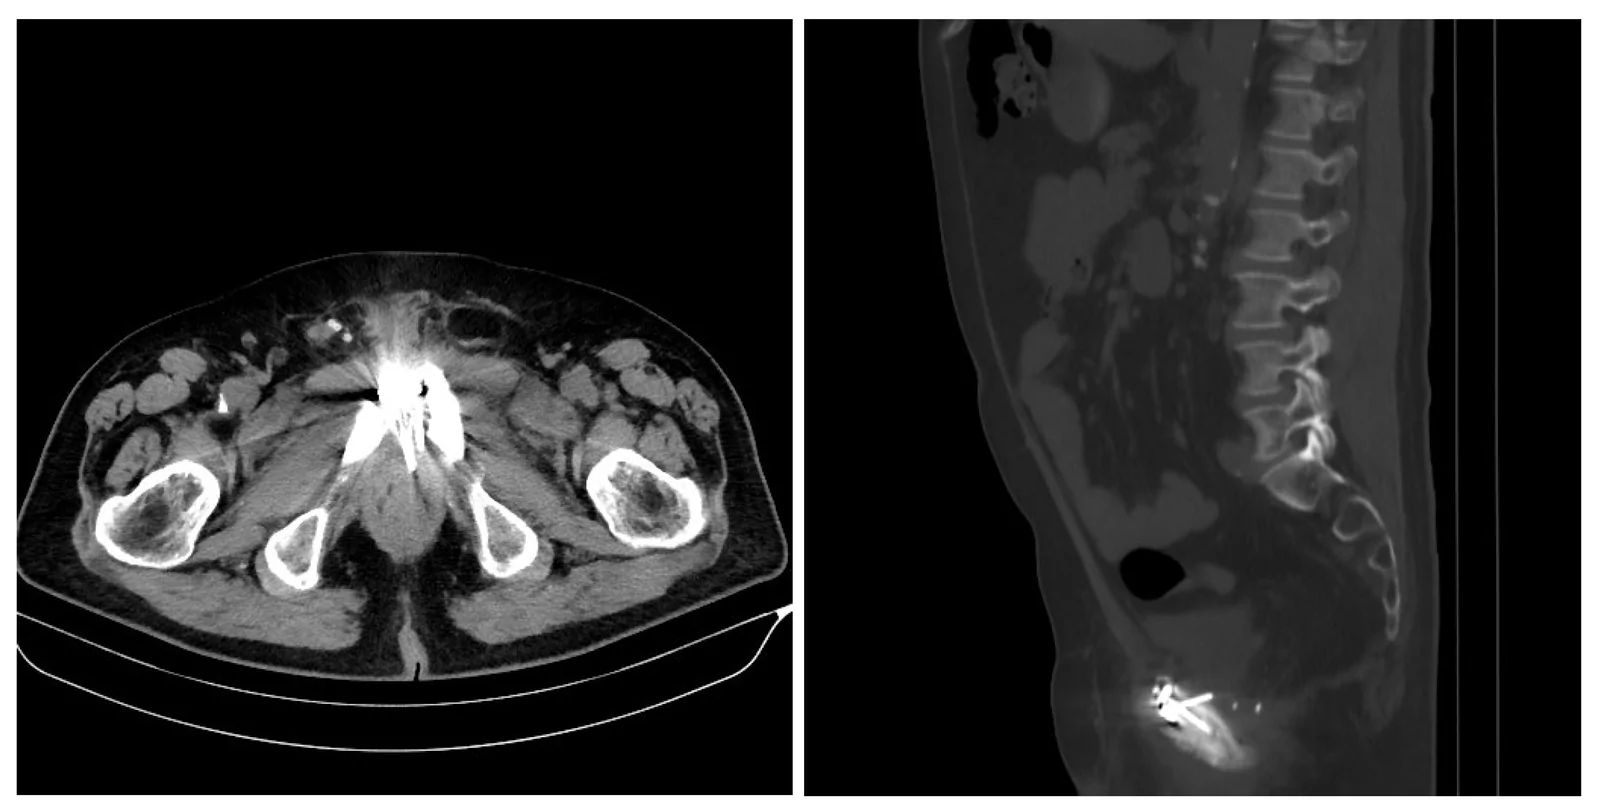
Contrast-enhanced computed tomography (axial and sagittal planes) demonstrating orthopedic osteosynthesis screws from previous pelvic fracture fixation projecting beyond the pubic bone into the adjacent pelvic soft tissues.
